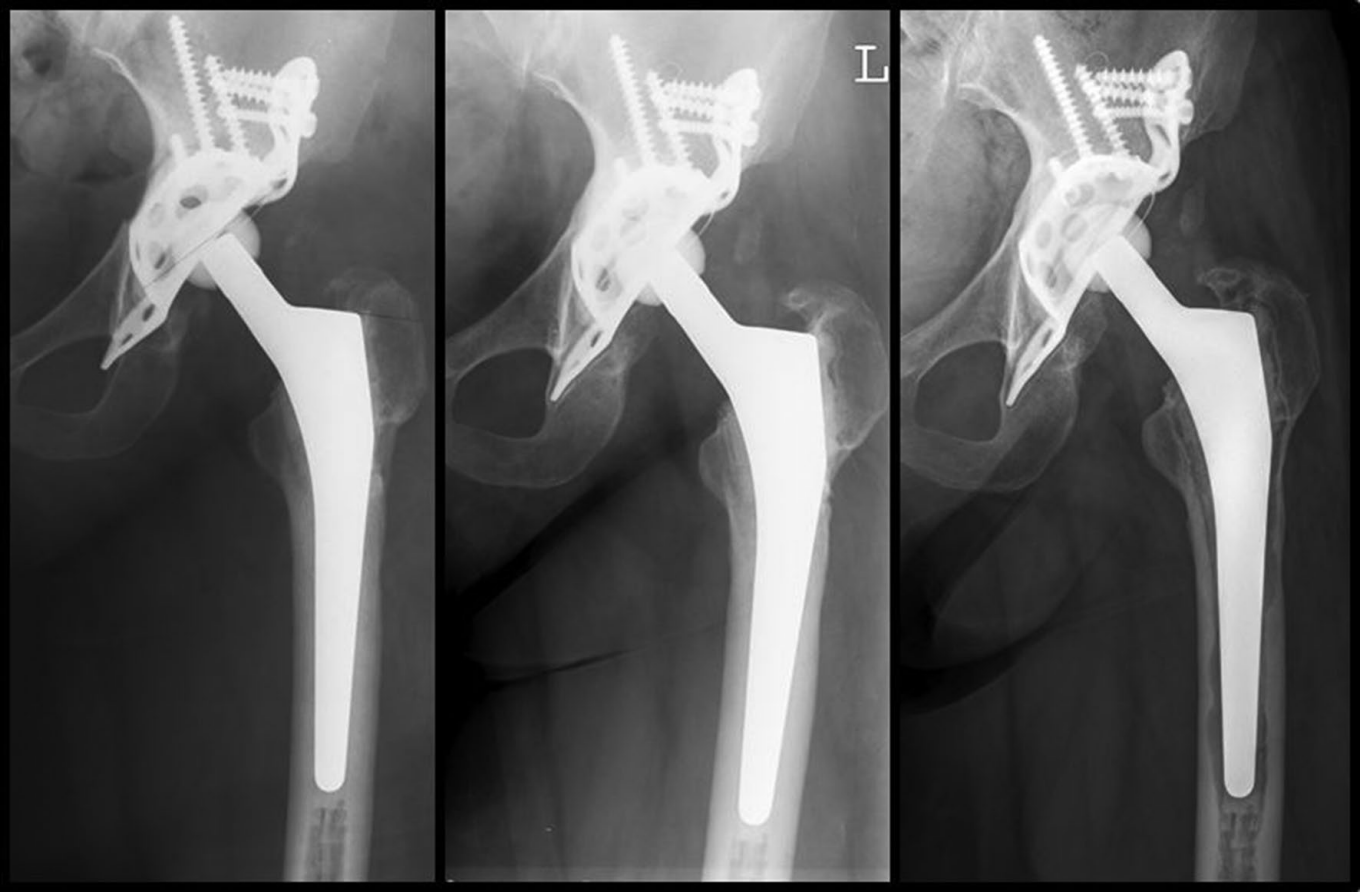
Cemented stem revision (Virtec stem and Burch-Schneider anti protrusion cage) of a 58-year-old woman, revision due to septic loosening. Preoperative defect Paprovsky grad 2. Radiographic follow-up postoperative (a), 10 years (b) and after 13 years (c) postoperative
